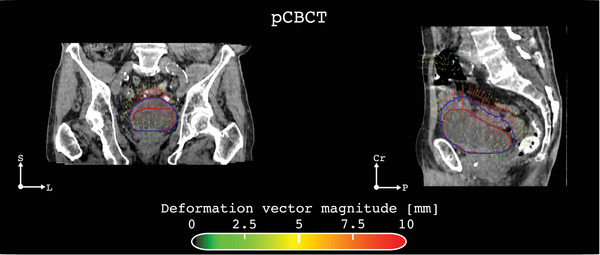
Case study patient for evaluating intra‐fractional bladder changes during CBCT‐guided online ART. Planning (red) and verification (blue) bladder contours are overlaid on the planning CBCT (pCBCT). The colored arrows indicate the direction and magnitude of anatomical changes during the on‐couch adaptation time. For this fraction, the time between pCBCT and vCBCT was 24 min.
